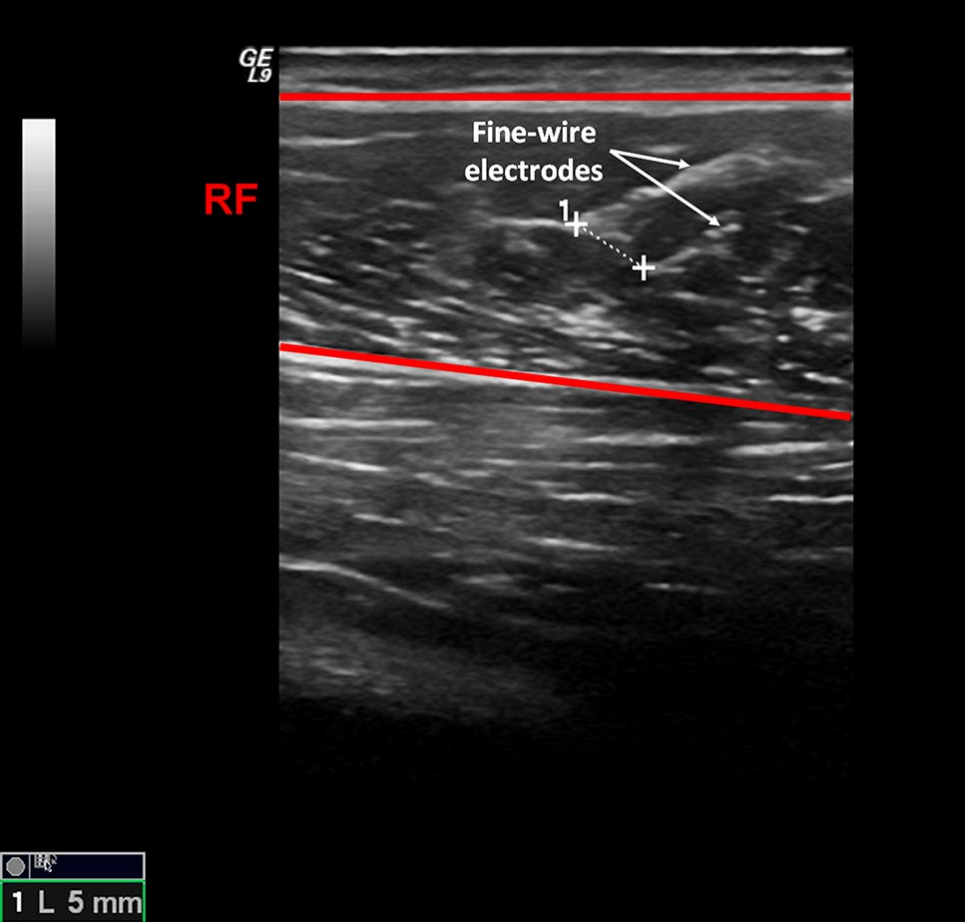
Ultrasound image acquired during fine-wire electrode insertion in the rectus femoris (RF) muscle (located between the *red lines*). The tips of the electrodes are marked with a *white cross*, indicating the inter-electrode distance (≈5 mm)
